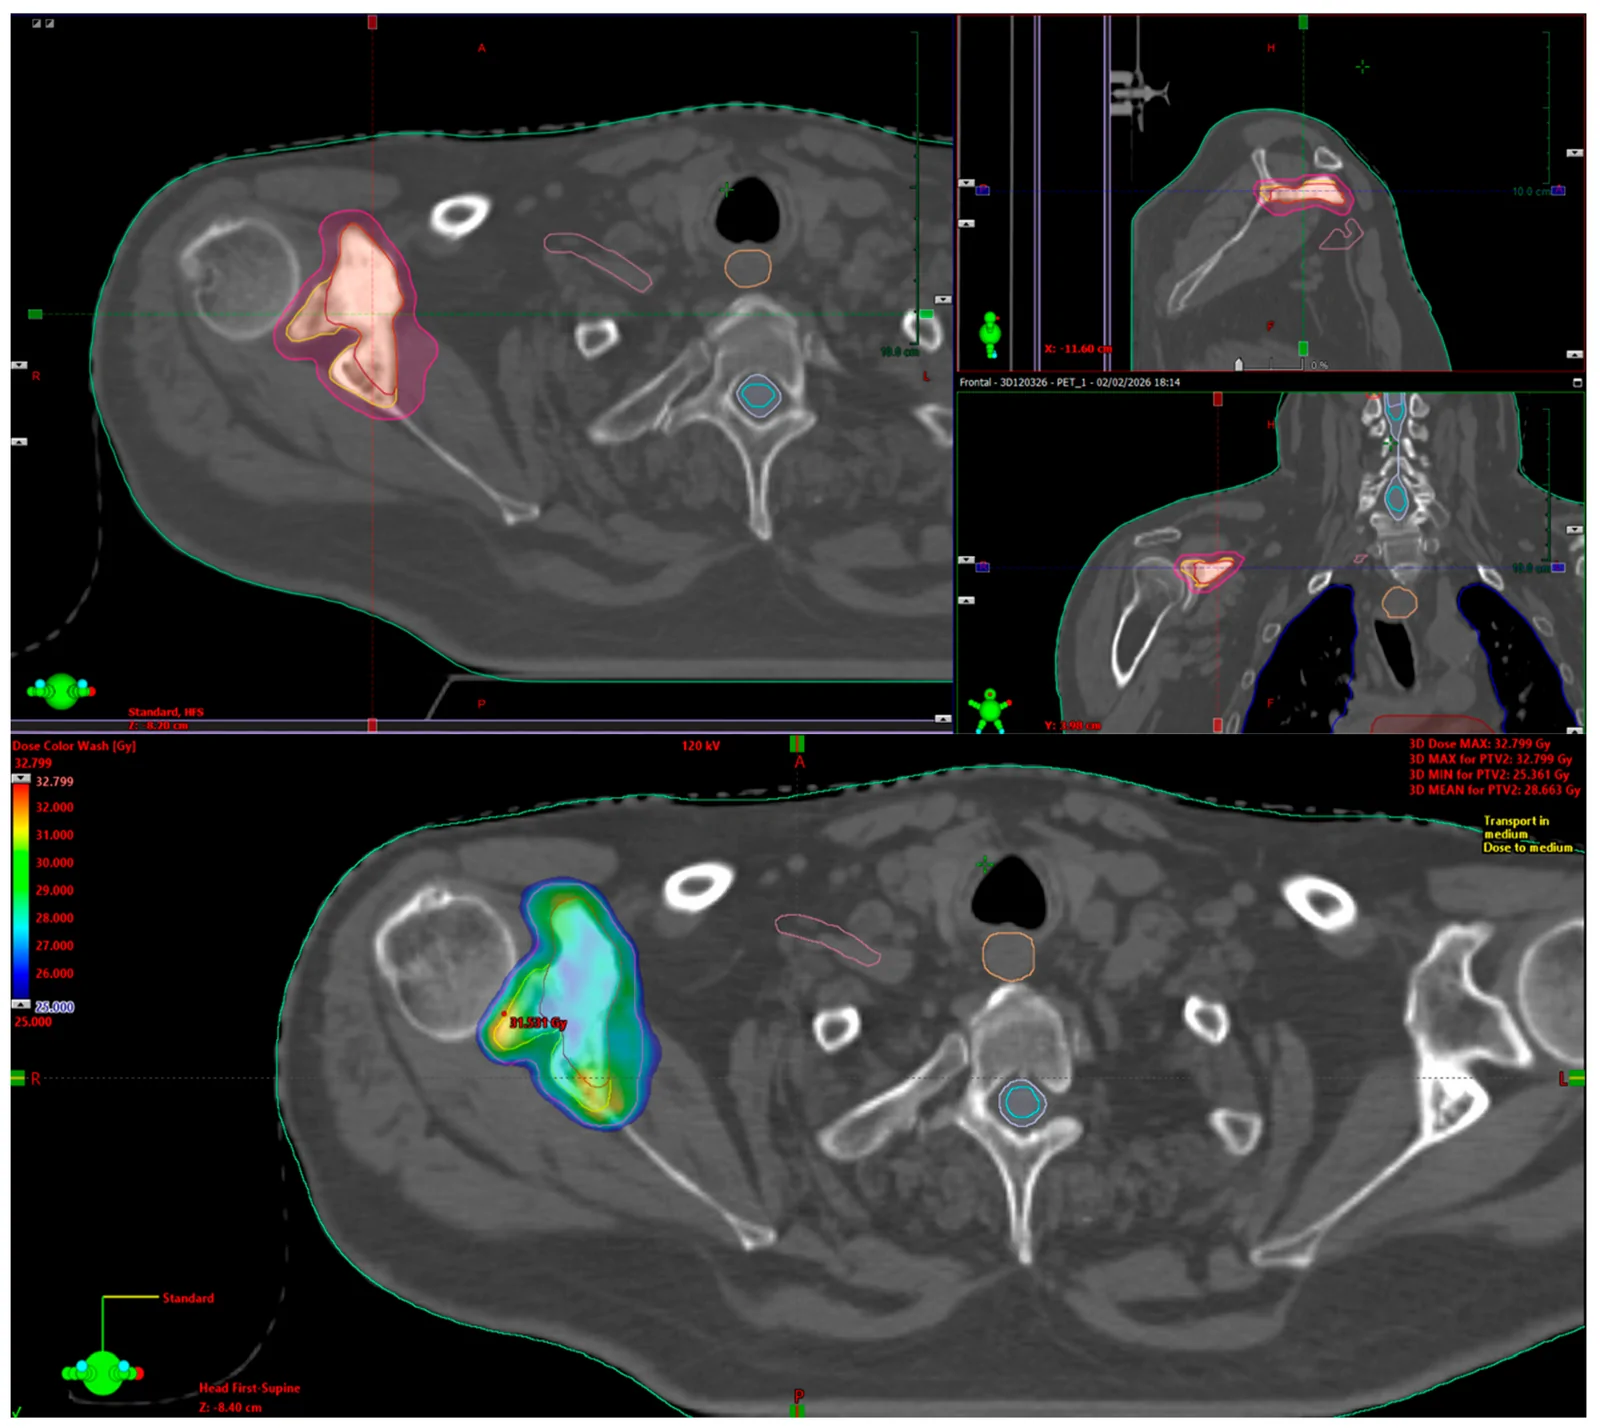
Treatment planning and dose distribution of SBRT directed to a PSMA-positive lesion in the coracoid process of the right scapula.
